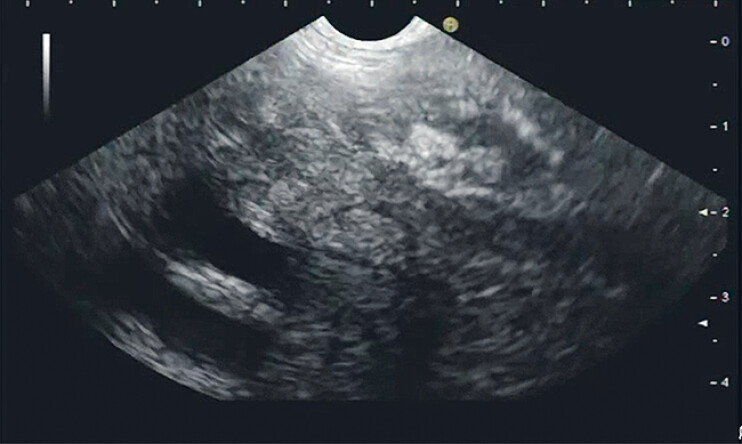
Transcolonic endoscopic ultrasound revealed a retroperitoneal, diffuse echo-reduced mass suggestive of retroperitoneal fibrosis.
